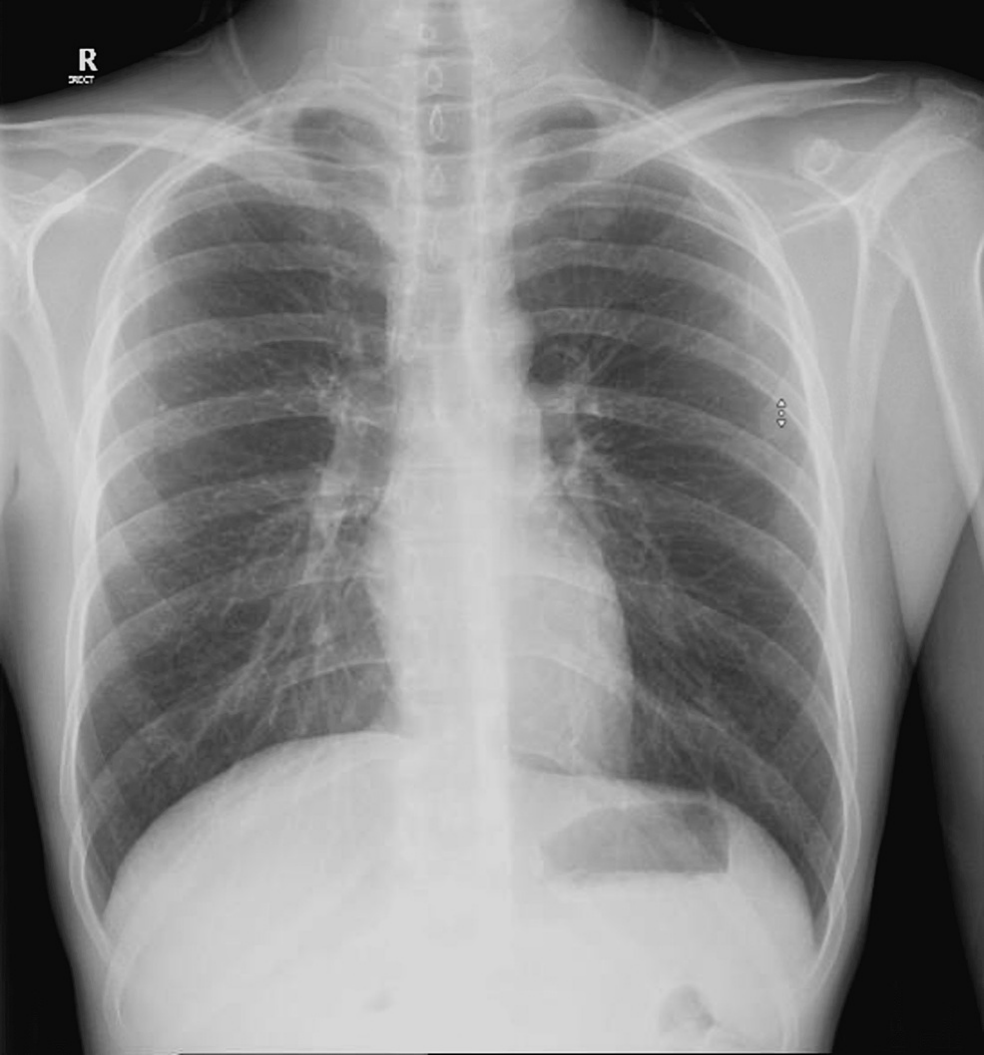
A posterior-anterior (PA) chest X-ray showing normal results with no evidence of tuberculosis in the lungs
